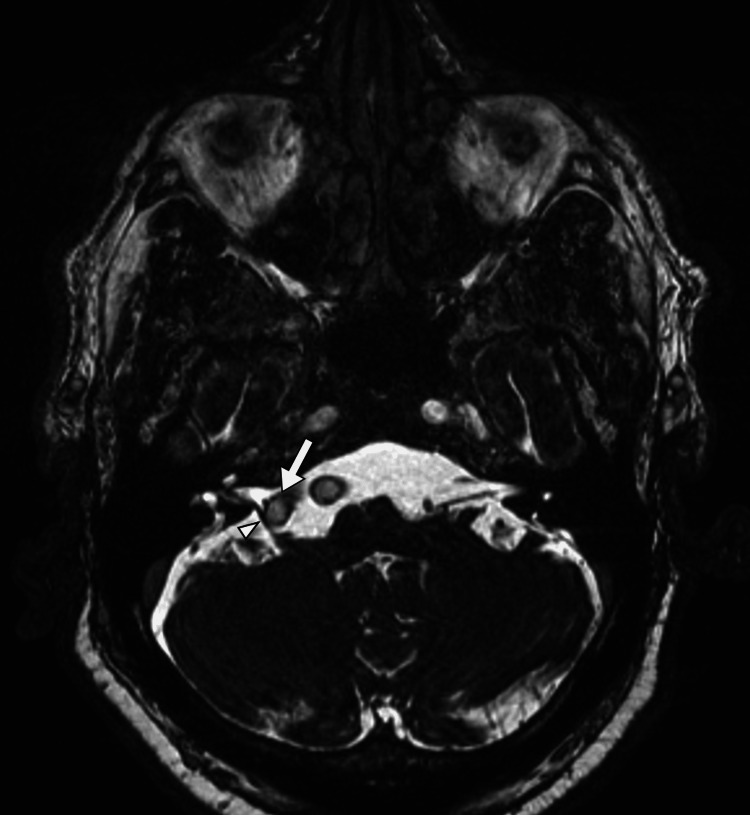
An axial image of the patient's brain MRI in the constructive interference of the CISS sequence shows the dilated right vertebral artery (arrow) compressing and displacing the right facial nerve (arrowhead).
